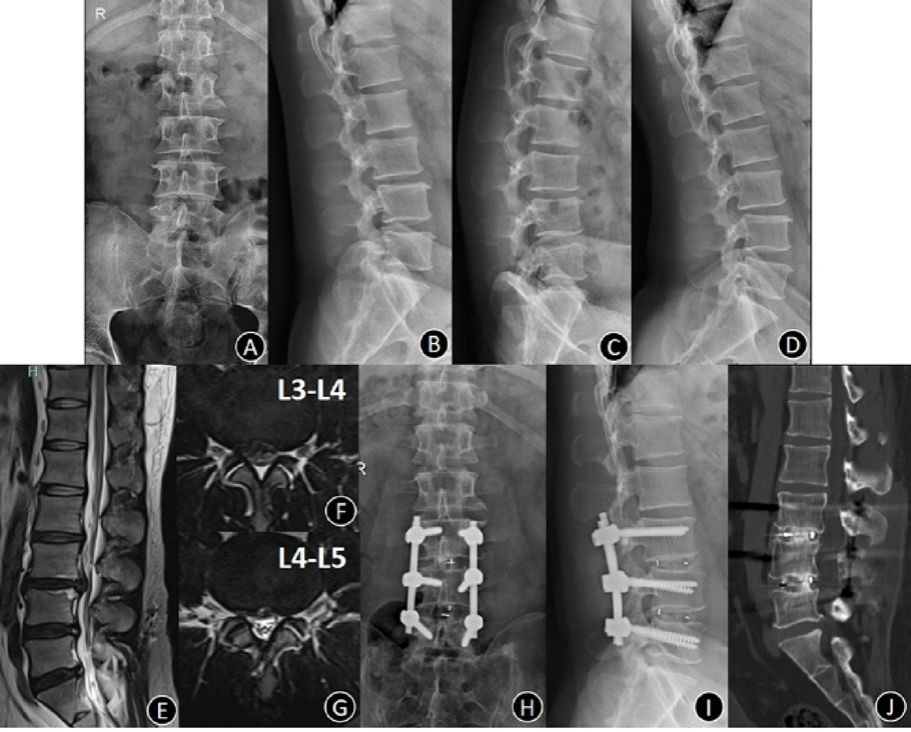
Bilateral pedicle screw fixation (BPSF) combined with intervertebral fusion (IF) to treat two-level lumbar degenerative disease. Male, 33 years old, complaining with lower back pain and radiating pain of left leg for two years. Preoperative diagnosis was L3-L5 lumbar disc herniation (LDH). (A)–(D) Anteroposterior, lateral, extension and flexion position plain indicated no instability and olisthy. (E) and (G) Preoperative MRI showed L3-L5 LDH; (H) and (I) Anteroposterior and lateral radiograph at 2 years after-surgery indicated that the location of internal fixation was good; (J) CT at 2 years after-surgery showed IF was excellent.
